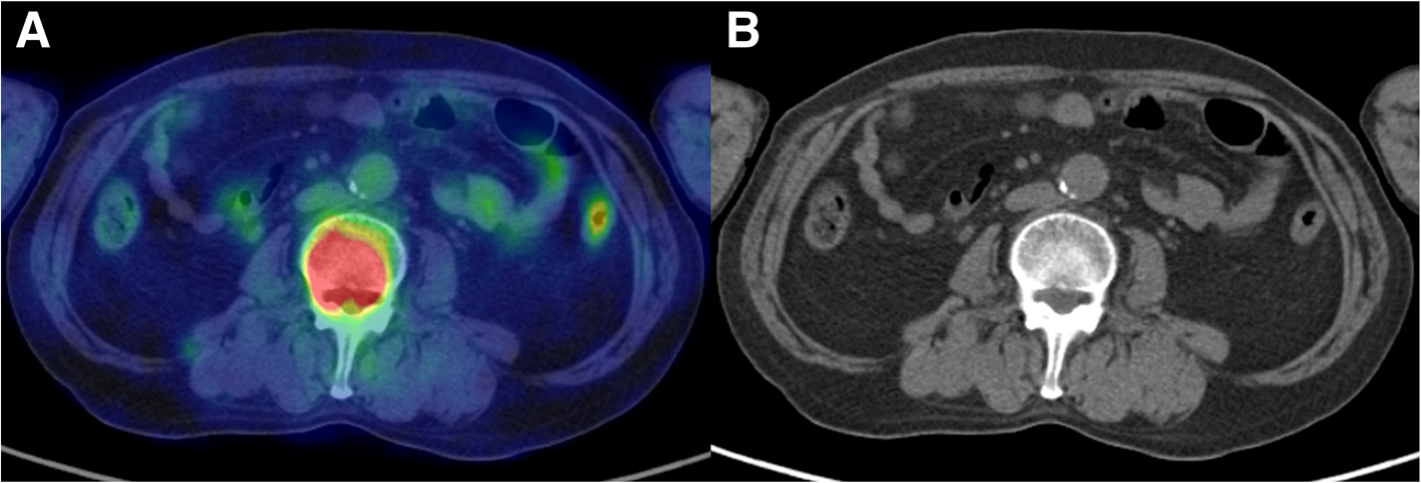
The PET/CT-positive and MDCT-negative case. Bone marrow involvement with obvious FDG uptake was detected by PET/CT (a). The MDCT scan showed no mass lesion (b)
